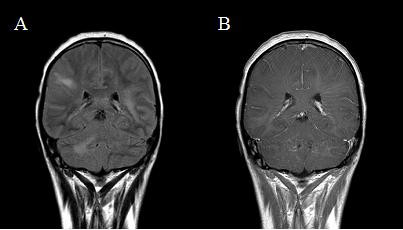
Magnetic resonance imaging (MRI) scans. This MRI scan from the patient's initial presentation shows a perivascular infiltration and intraparenchymal, large-scale lesions in both hemispheres on the T2-weighted images (A). Application of gadolinium revealed no enhancement of the lesions in the T1-weighted images (B).
